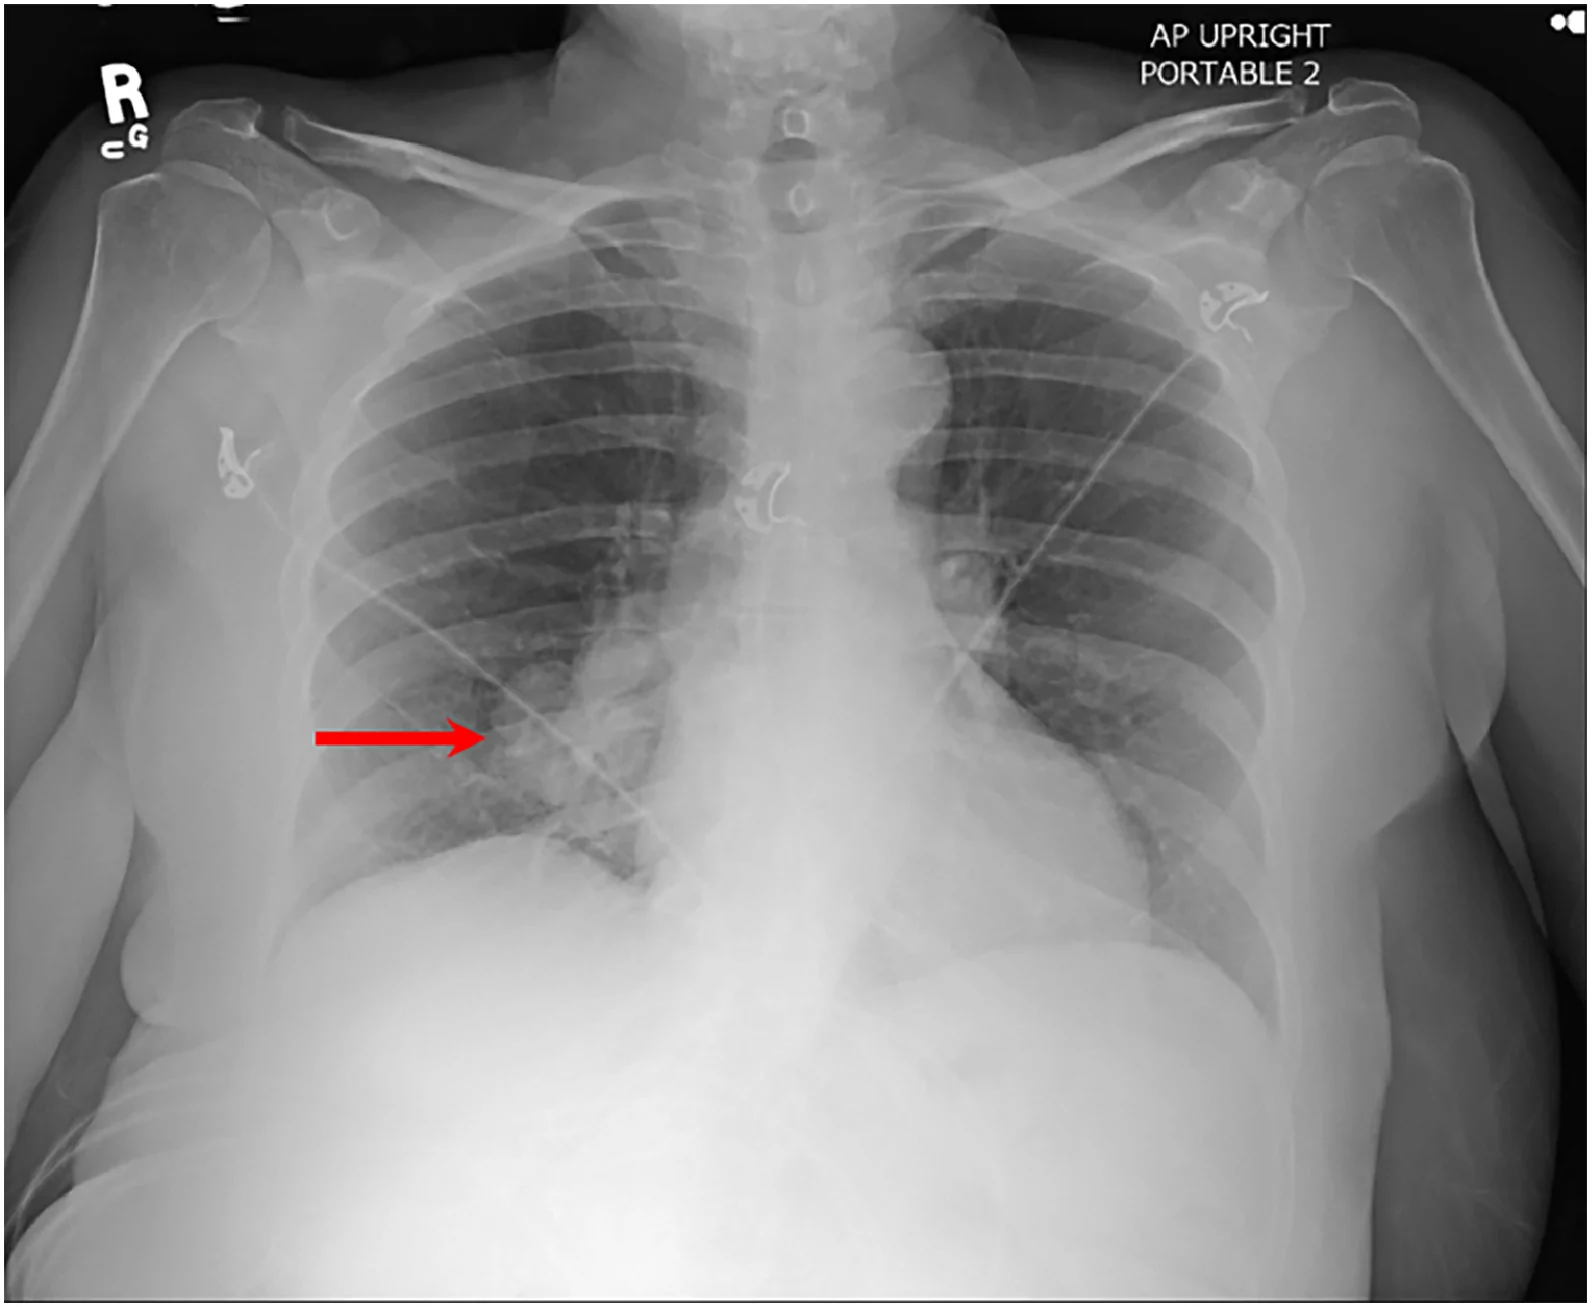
AP radiograph of the chest demonstrates a right infrahilar mass (arrow).
